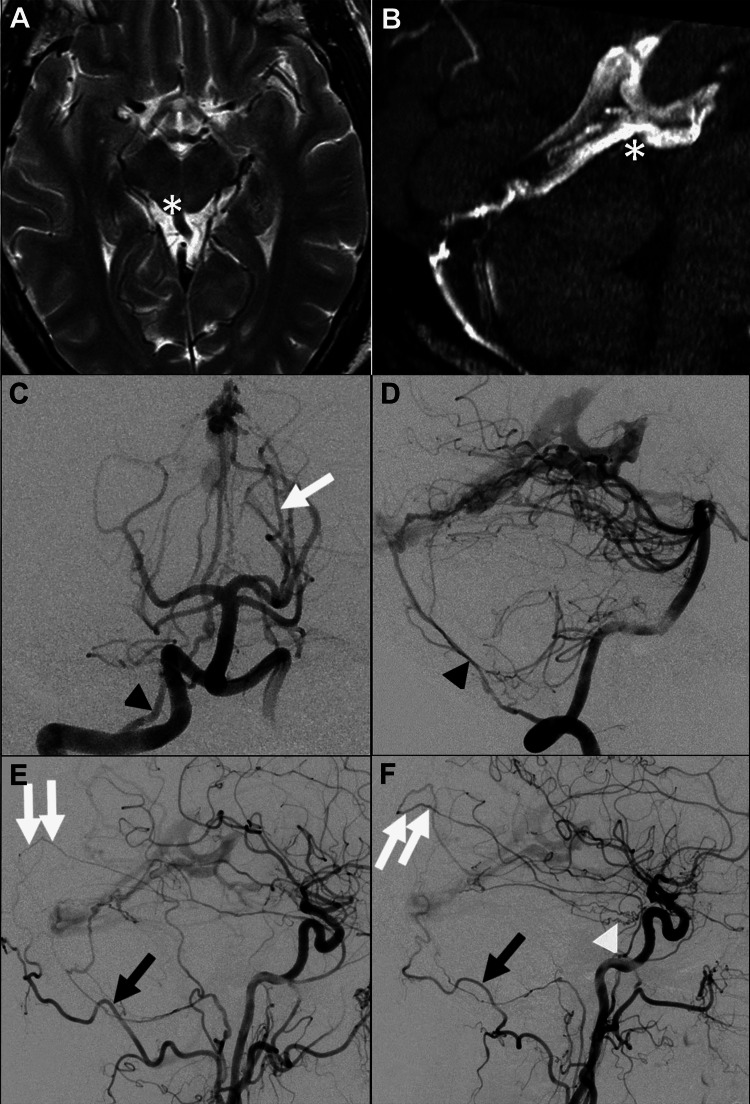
MRI and angiography of an enlarged cerebellar vein and deep vessels, with a tentorial dural arteriovenous fistula draining into the straight sinus. A) Axial T2-weighted MRI demonstrates a significantly enlarged cerebellar vein (indicated by a white asterisk) along with abnormally prominent deep vessels around the tentorium; B) sagittal maximum intensity projection MR angiography showing a significantly enlarged cerebellar vein (marked with a white asterisk); C) digital subtraction angiography (DSA) of the right vertebral artery, anteroposterior view, shows the tentorial dural arteriovenous fistula (DAVF) receiving blood supply from the right posterior meningeal artery (indicated by a black arrowhead); D) lateral view of the common carotid artery, indicating the left artery of Davidoff and Schechter (white arrow); E) lateral view of the left vertebral artery showing blood supply from the left and right occipital arteries (black arrows); F) digital subtraction angiography, anteroposterior and lateral views of the right external carotid artery, reveals the DAVF’s additional blood supply from the left and right middle meningeal arteries (represented by double white arrows), and tentorial branches from the right ICA (highlighted by a white arrowhead). The fistula drains into the straight sinus through the vein of Galen and retrograde outflow is observed in the superior and inferior sagittal sinuses and the deep venous system.
